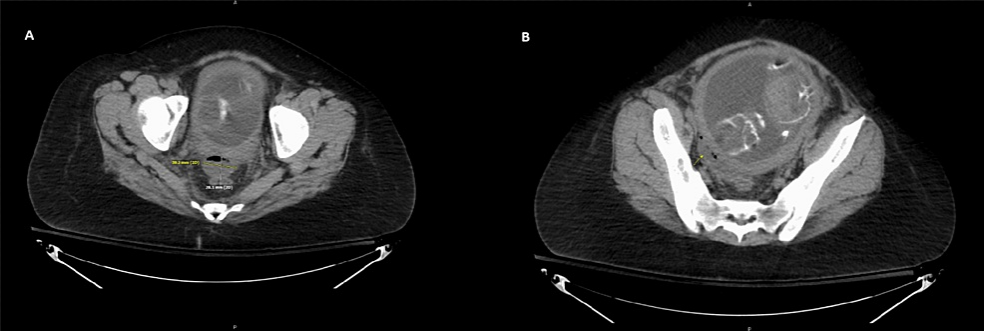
Confirmatory CT Imaging without Contrast of Sigmoid Diverticular Abscess CT scans used dose modulation, iterative reconstruction, and/or weight-based dosing to reduce radiation dose to as low as reasonably achievable. Impression: A: 2.8 x 3.9 cm fluid and gas collection within the rectal pouch of Douglas tracking along the right posterior lateral and superior uterus. B: Sigmoid colonic inflammatory changes and ascites when compared to previous imaging. Prominent gas and stool-filled diverticulum noted extending along the sigmoid antimesenteric border. No gross pneumoperitoneum.
